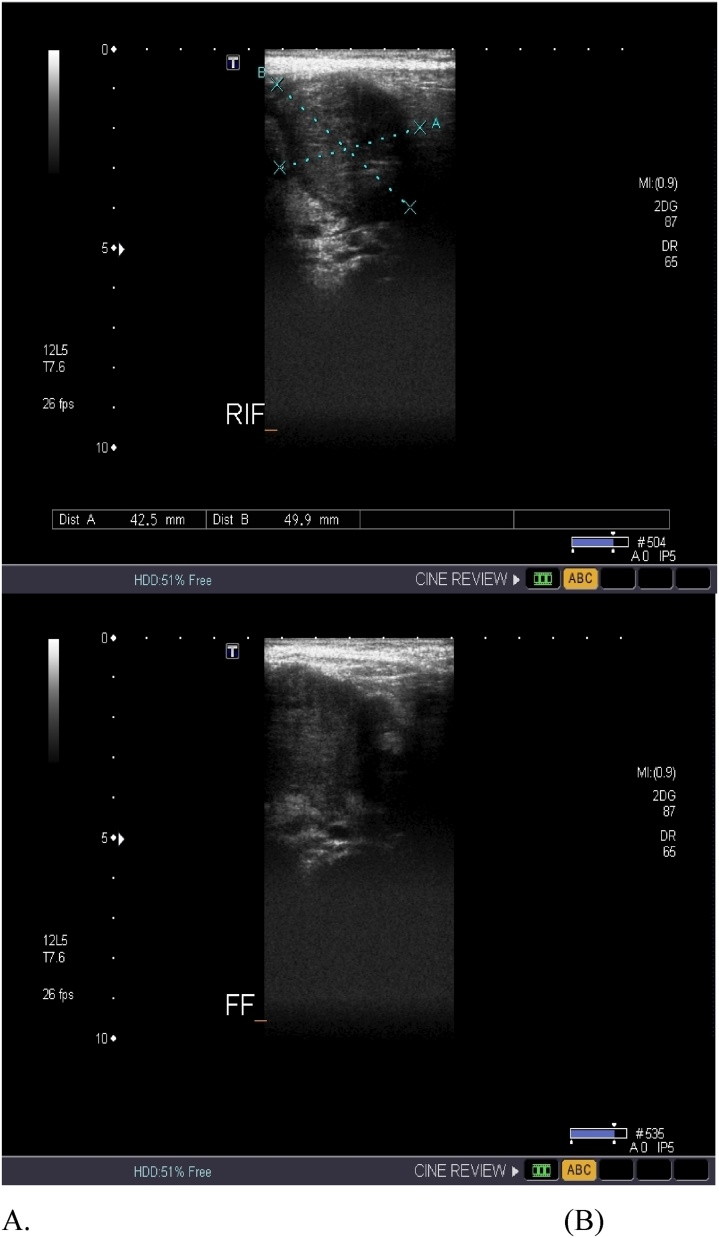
A) Abdominal ultrasound showing appendicular mass. B) Abdominal ultrasound showing fluid collection.
